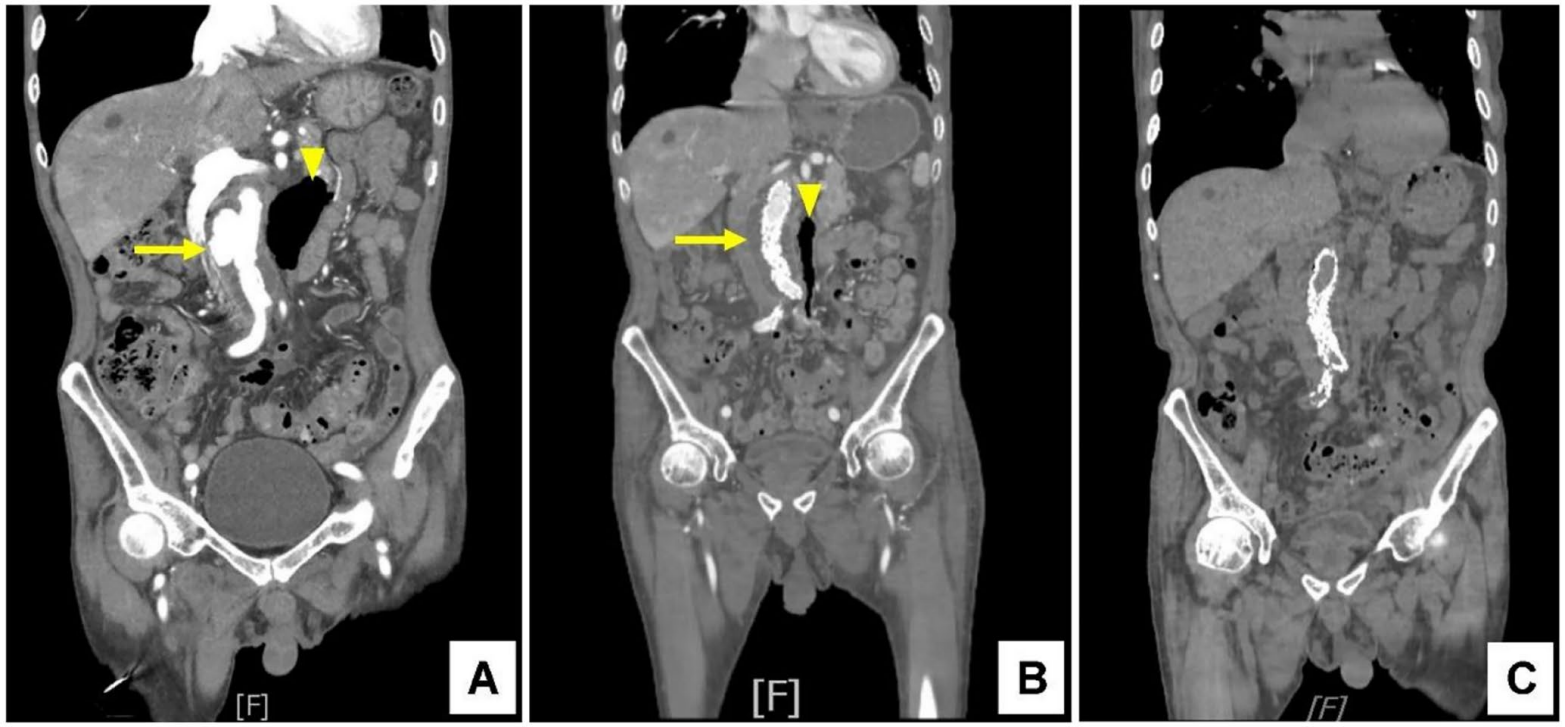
Serial contrast‐enhanced computed tomography angiography (CTA) of the abdomen. (A) Preoperative coronal CTA demonstrates an infrarenal abdominal aortic aneurysm (AAA) with an irregular aneurysmal contour (yellow arrow). Adjacent para‐aortic inflammatory changes with gas‐containing collections are noted (yellow arrowhead), consistent with infectious aortitis, likely originating from contiguous sigmoid diverticulitis. (B) Post‐percutaneous endovascular aneurysm repair (PEVAR) coronal CTA shows successful exclusion of the aneurysmal sac with transformation of the AAA into a thrombosed pseudo‐lumen (yellow arrow). Marked interval reduction of the adjacent diverticulitis‐associated gas cavity and para‐aortic inflammatory changes is observed (yellow arrowhead). (C) Follow‐up coronal CTA demonstrates favorable recovery, with stable stent graft position, no evidence of endoleak, and near‐complete resolution of periaortic inflammation and gas collections, indicating good post‐treatment response.
